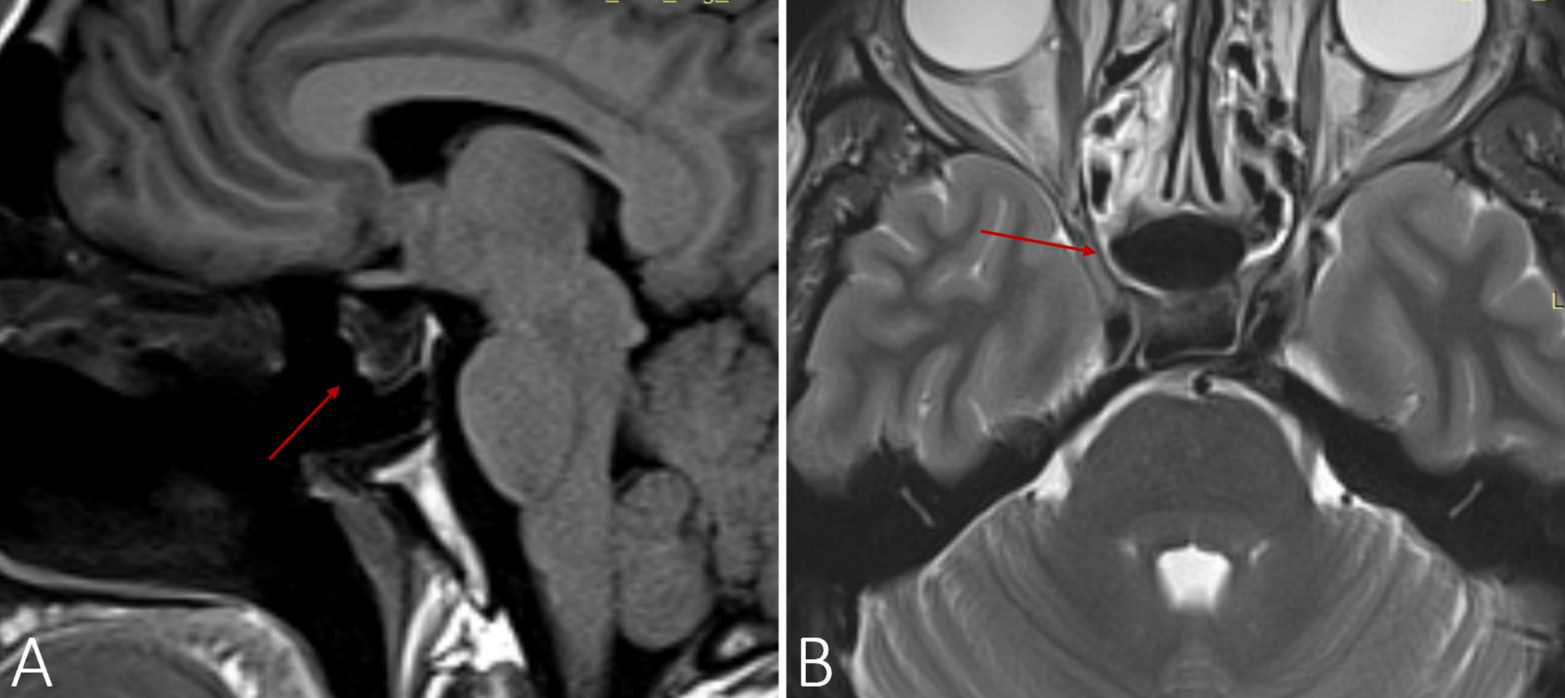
Cranial MRI: T1-weighted saggital plane (A) and T2-weighted axial plane (B) one year after transspnehoidal hypophysectomy, demonstrating an empty sella (arrow) and a confirming absence of new expansive lesions in the sellar region.
